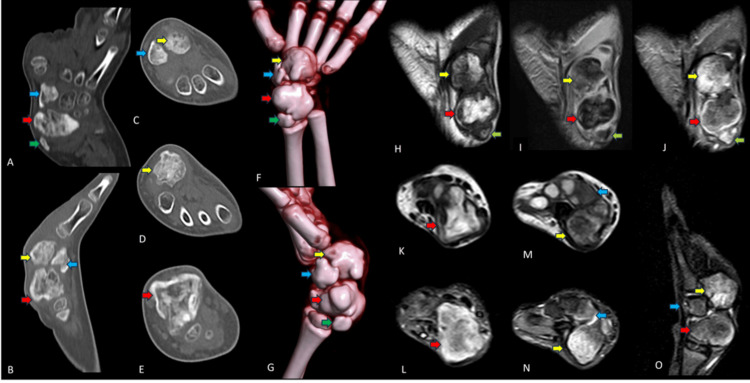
CT images in (A) coronal oblique, (B) sagittal reformatted, (C, D) axial sections at the level of the distal carpal bones, and (E) axial section at the level of the proximal carpal row demonstrate expansile sclerotic lesions in the trapezium (blue arrow), trapezoid (yellow arrow), scaphoid (red arrow), and along the inferolateral margin of the scaphoid (green arrow). Volume-rendered images show similar lesions in (F) AP and (G) lateral views. MRI coronal images depict hyperintense cartilage caps in the trapezoid (yellow arrow), scaphoid (red arrow), and along the abductor pollicis longus tendon (green arrow) in (H) T1-weighted, (I) T2-weighted, and (J) fat-suppressed T2-weighted sequences. Axial images at the level of the proximal carpal row show scaphoid lesions (red arrow) in (K) T1-weighted and (L) fat-saturated T2-weighted sequences. At the level of the distal carpal row, lesions are seen in the trapezium (blue arrow) and trapezoid (yellow arrow) in (M) T1-weighted and (N) fat-saturated T2-weighted axial sections. (O) Sagittal fat-saturated STIR image demonstrates lesions in the trapezium (blue arrow), trapezoid (yellow arrow), and scaphoid (red arrow).
